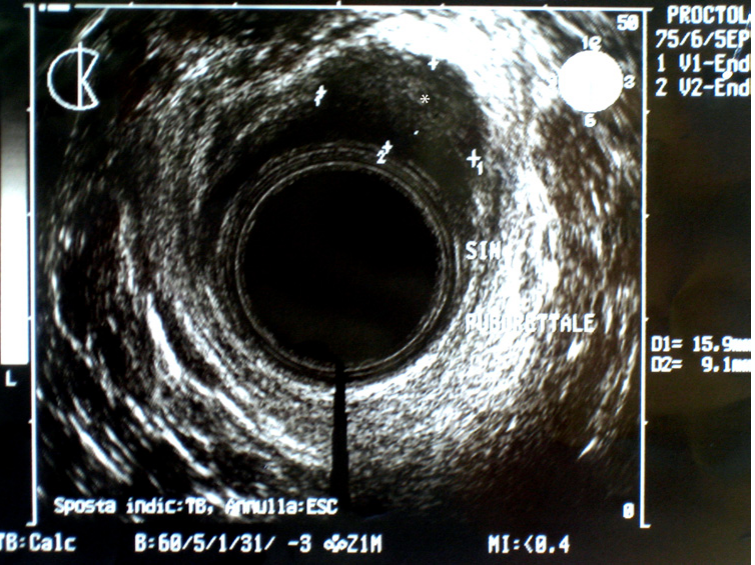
Endoanal ultrasonography (transverse plain) shows the mass (*) located along the left anterior aspect of the anal canal.
